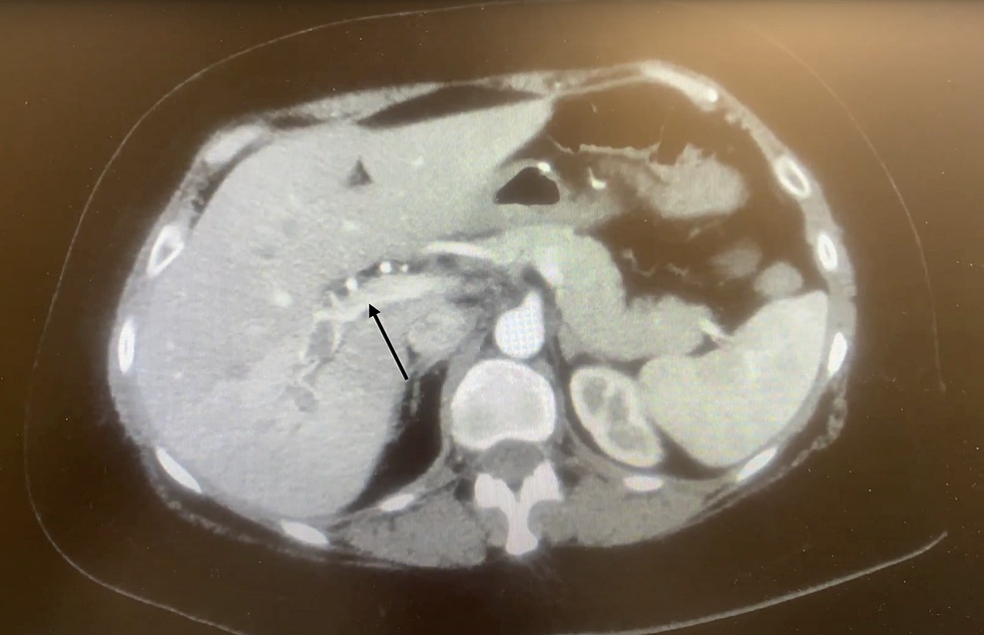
CT scan showing hyperattenuation of the portal vein lumen (arrow) suggestive of PVT and resultant differential enhancement of the liver PVT - portal vein thrombosis
